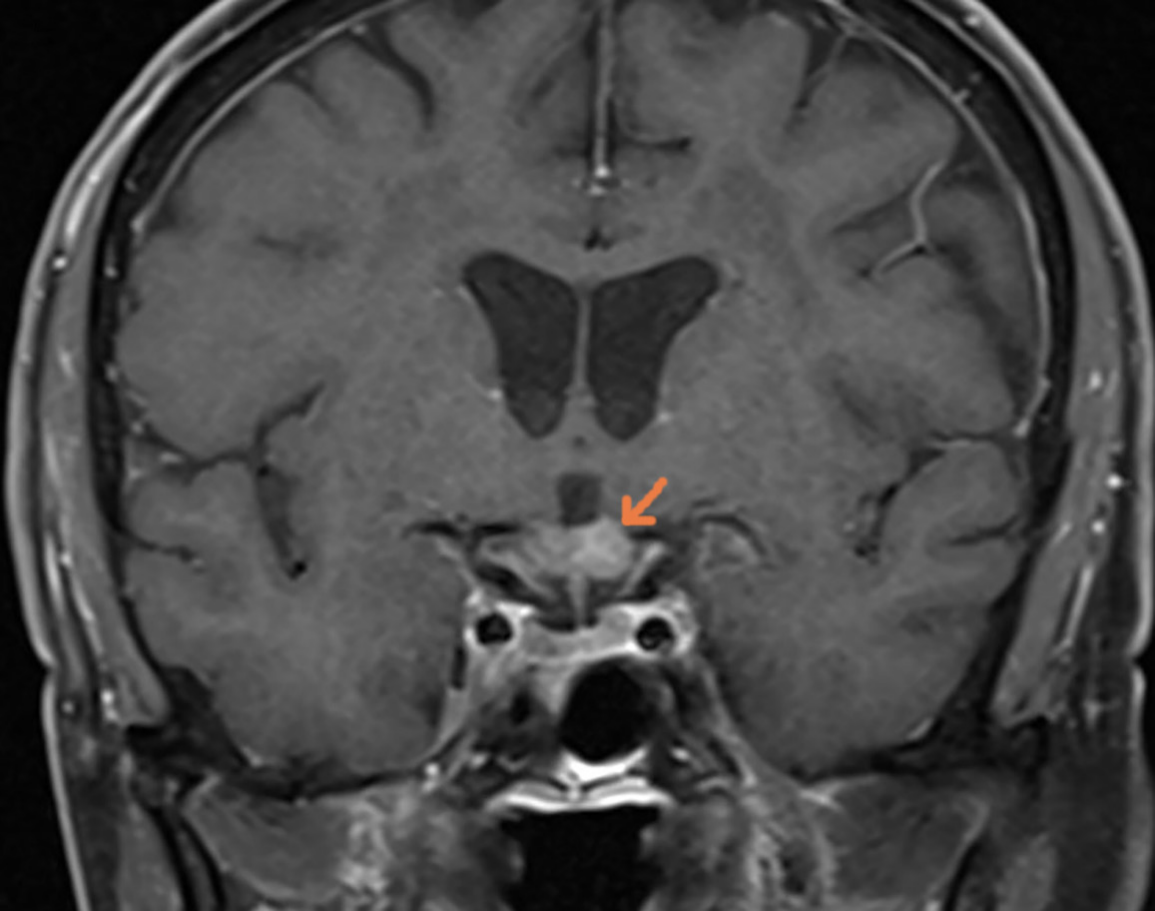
Preoperative MRI, coronal T1 with contrast, showing the lesion of the chiasma.
